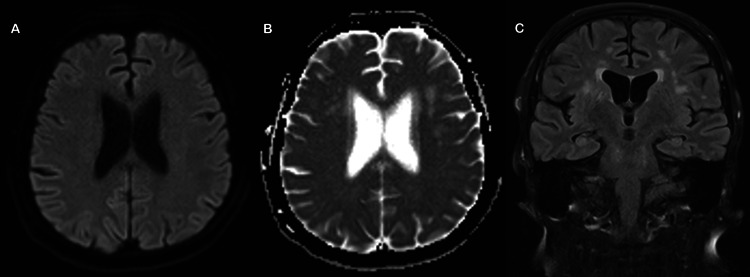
Initial MRI performed in the emergency department. Findings shown: A) DWI axial demonstrates no acute infarction; B) apparent diffusion coefficient (ADC) axial shows chronic ischaemic changes; and C) fluid-attenuated inversion recovery (FLAIR) coronal shows similar ischaemic changes.
